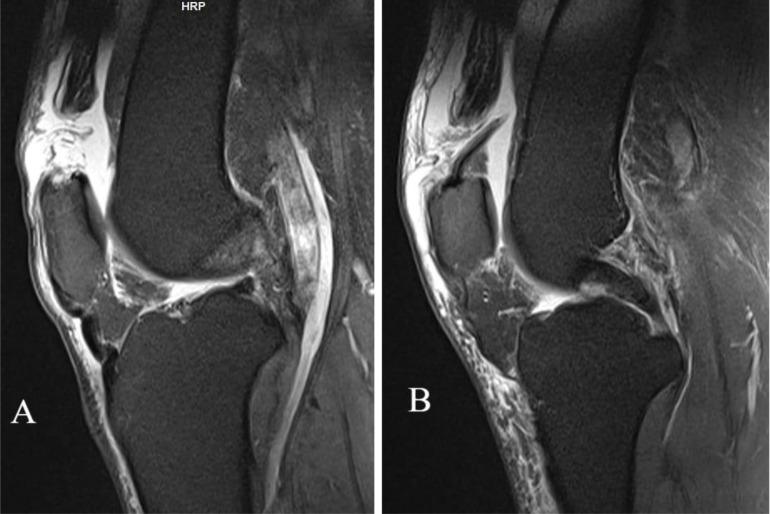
MRI knee showing bilateral rupture of the quadriceps tendon, as there is a noticeable increase in signal intensity on both sides along with loss of fiber continuation. (A) Right knee MRI; (B) left knee MRI.
